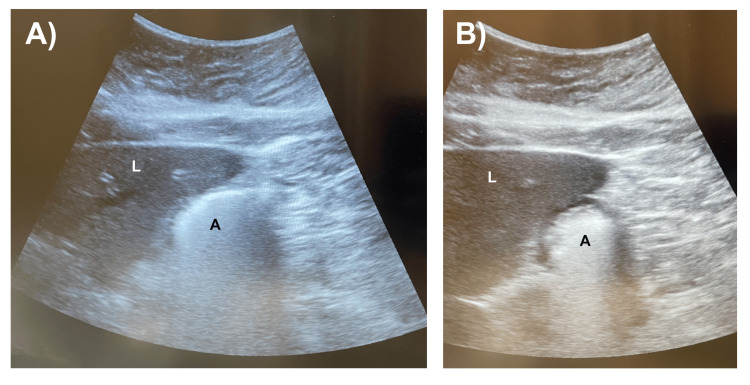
Gastric point-of-care ultrasound demonstrating solid gastric contents in the antrum in the right lateral decubitus position (A) and supine position (B) of the same patient Pertinent structures were identified, such as the liver (L) and gastric antrum (A). Solid gastric contents appear heterogeneous and hyperechoic (bright) in the antrum. Structures distal to the solid contents (A, B) may not be visible due to poor penetration of the ultrasound waves, also known as “shadow artifact.”
